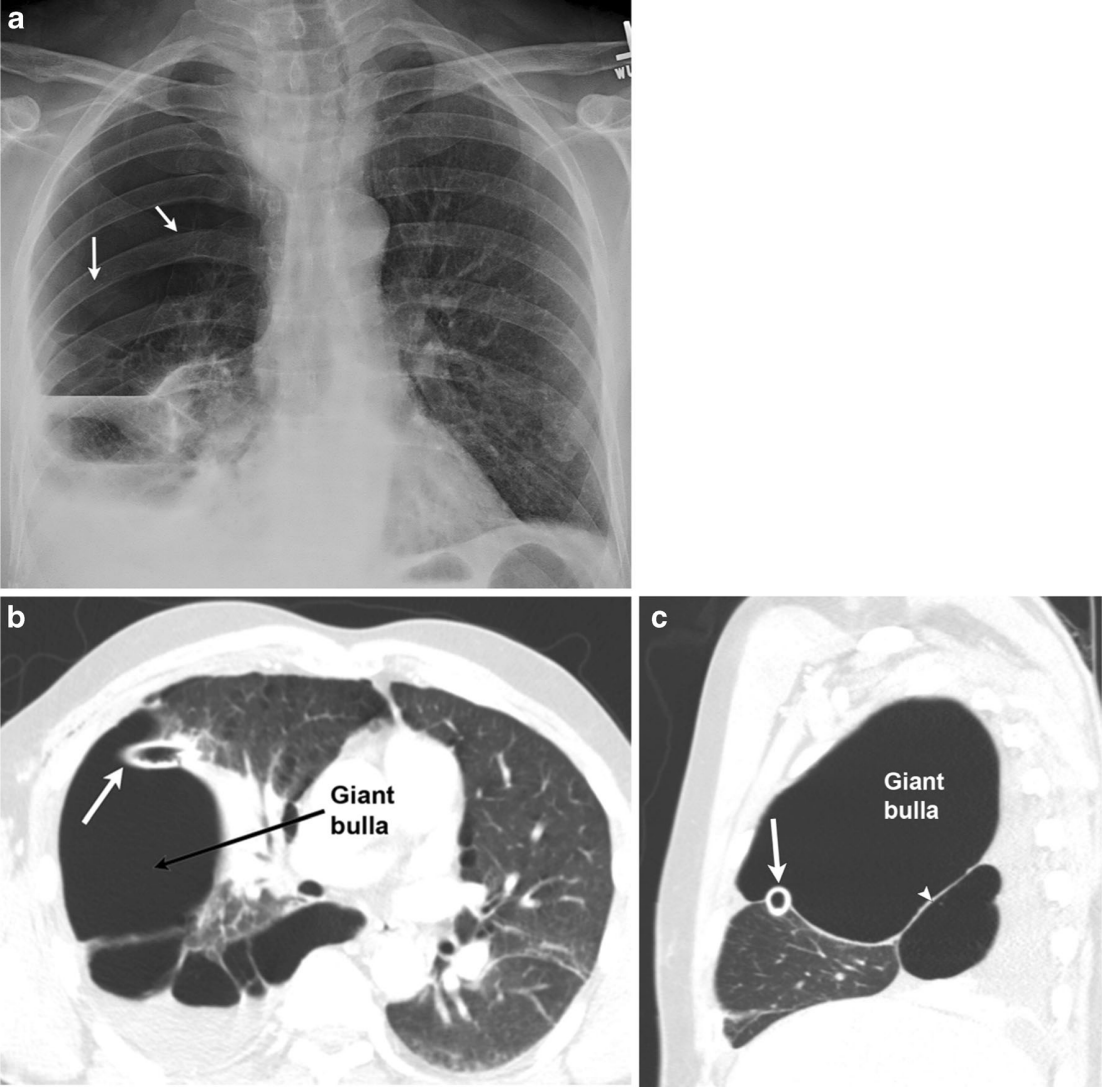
A 46-year-old smoker presenting to the emergency department (ED) with chest pain. Frontal chest radiograph (a) demonstrates hyperlucency through the right chest (*thin arrows*) with few dependent fluid levels; this was interpreted as a hydro pneumothorax. Post chest tube placement, both axial (b) and sagittal (c) CT images demonstrate chest tube within the right minor fissure (*solid white arrow*), abutting a giant bulla (defined as bulla occupying more than 30 % of the hemithorax). The bulla can be differentiated from a pneumothorax by the presence of septae (*arrowhead*) and compression of the lung parenchyma, unlike a pneumothorax where a visceral pleural line should be seen. The initial radiographic assessment was therefore inaccurate
